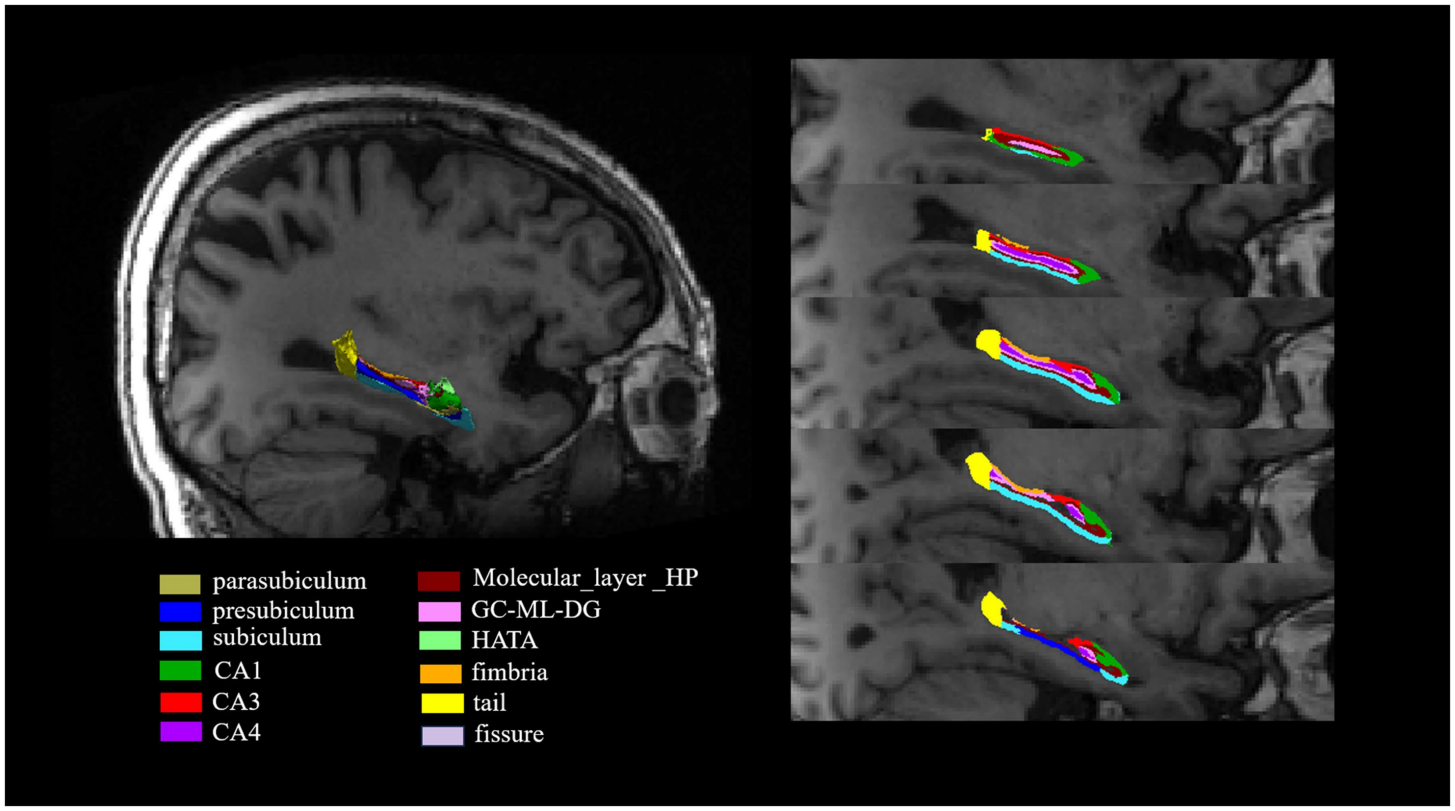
The segmentation of hippocampal subfields on T1-weighted MRI images. The hippocampus was segmented into the following subfields: parasubiculum, presubiculum, subiculum, CA1, CA3, CA4, molecular_layer_HP, GC-ML-DG, HATA, fimbria, tail and fissure. CA, Cornu ammonis; GC-ML-DG, Granule cell and molecular layer of the dentate gyrus; HATA, Hippocampus-amygdala transition area.
